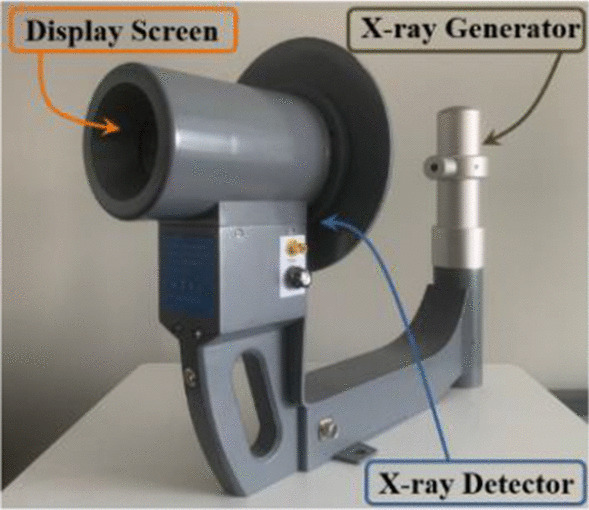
The portable X-ray machine
